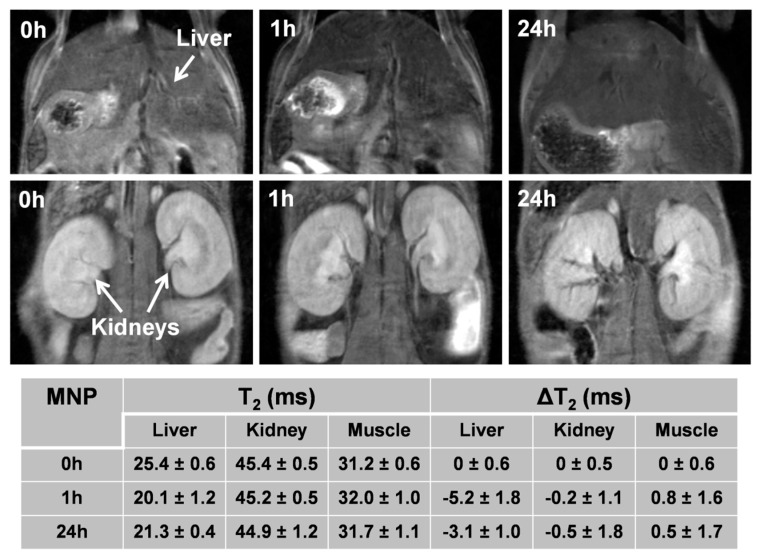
Representative*T*_2_-weighted MR images at different experimental times after the intravenous injection of PEGylated CF@MFNPs of liver (top) and kidneys (bottom). *T*_2_ and Δ*T*_2_ values of liver, kidneys and muscle at different times after the intravenous injection of PEGylated CF@MF NPs. Values correspond to the mean ± standard deviation (*n* = 3).
